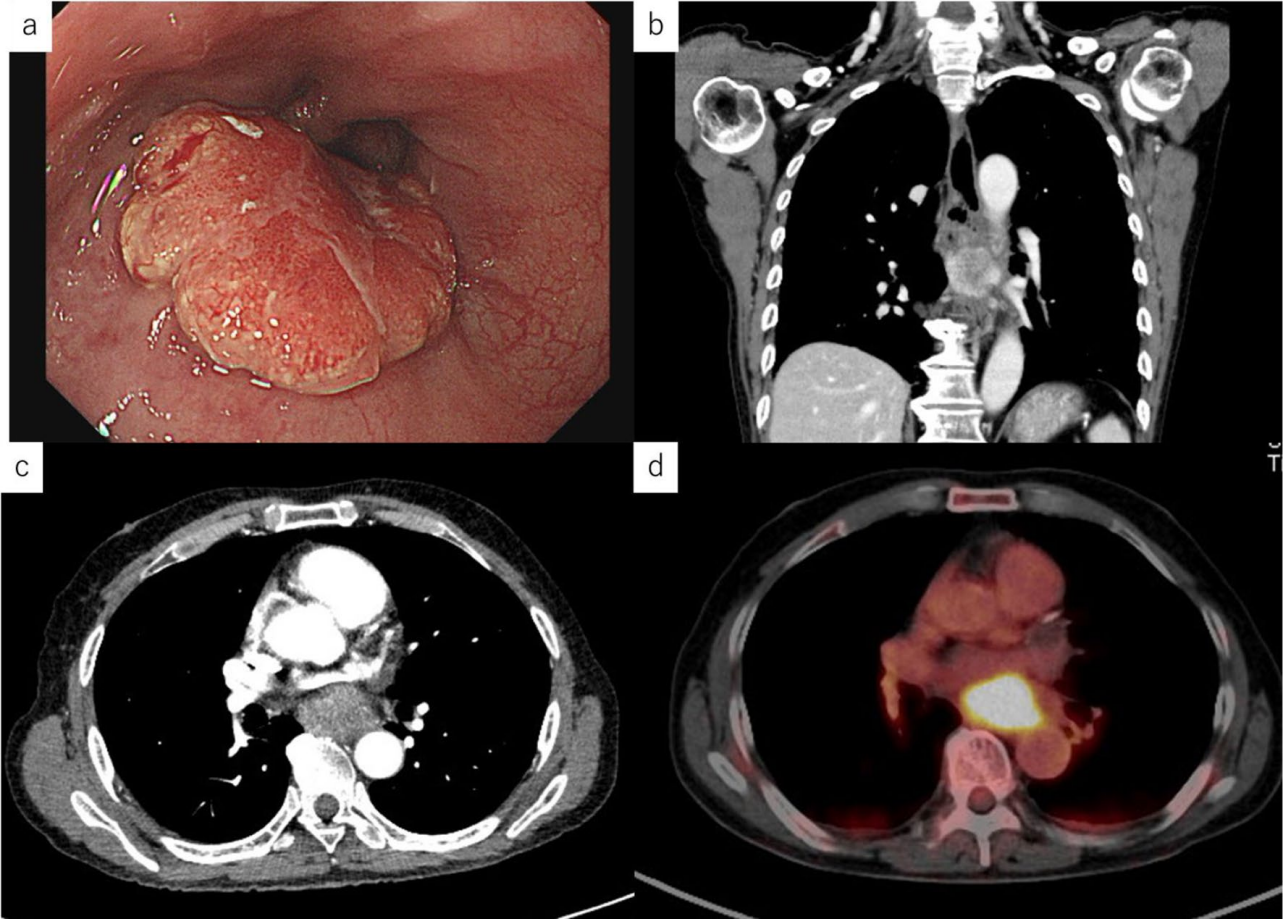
Test results of the patient. a Upper gastrointestinal endoscopy showing a type 2 tumor on the posterior wall of the mid-thoracic esophagus, approximately 29–32 cm from the incisor. b, c Contrast-enhanced computed tomography revealing a circumferential wall thickening in the mid-thoracic esophagus without enlarged lymph nodes or distant metastasis. d Positron emission tomography–computed tomography showing accumulation in the primary tumor, but no evidence of lymph node metastasis or distant metastasis
